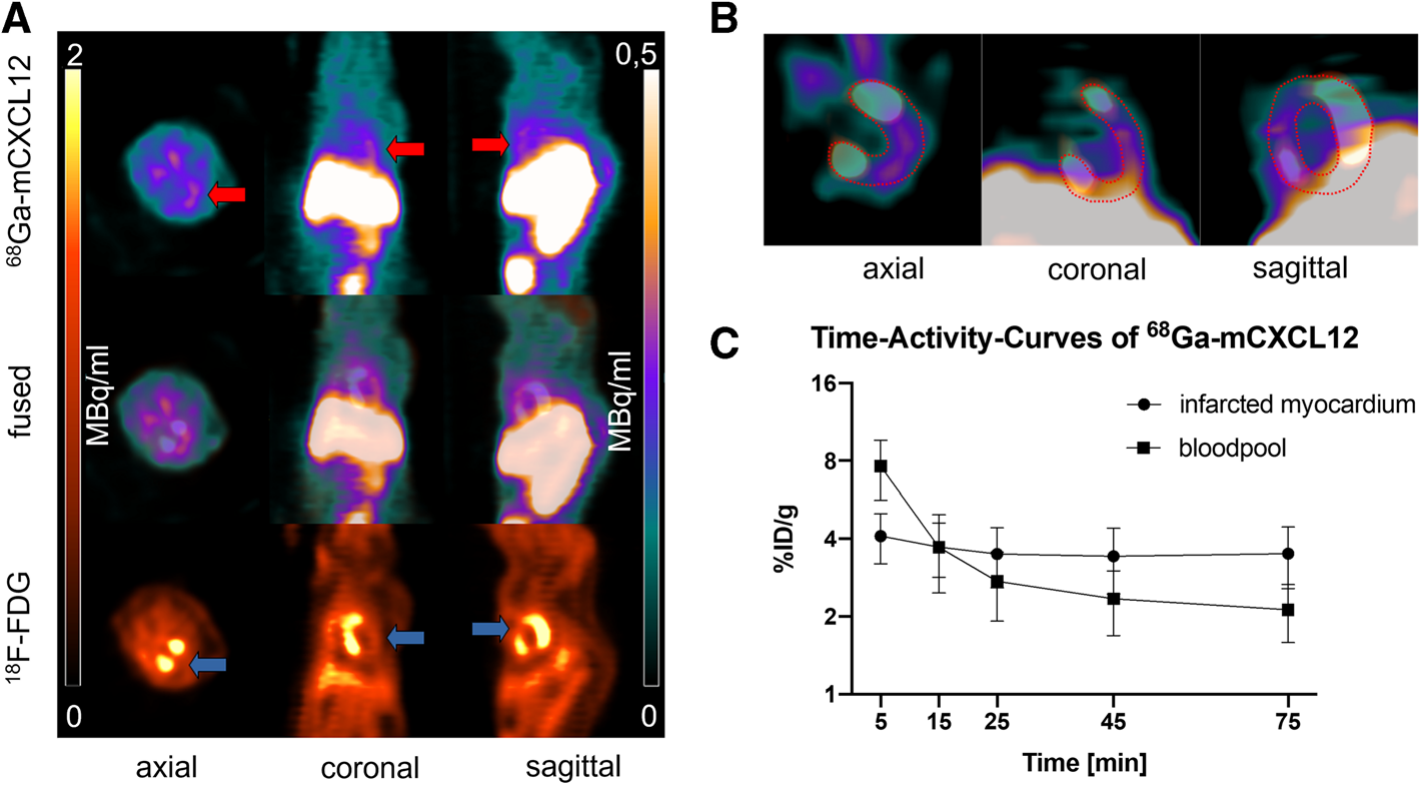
^68^Ga-mCXCL12 PET and time-activity curves. (A) PET image on day 3 with increased ^68^Ga-mCXCL12 uptake in the infarcted myocardium (red arrow: ^68^Ga-mCXCL12 uptake; blue arrow: infarcted myocardium). ^18^F-FDG PET showing the viable myocardium. (B) Fused and zoomed images of ^68^Ga-mCXCL12 and ^18^F-FDG PET with red dotted lines around the myocardium. (C) Representative ^68^Ga-mCXCL12 PET time-activity curves in the myocardium and the blood pool with the best infarct-to-blood pool ratio at the end of the acquisition time. All data represent mean ± SEM
